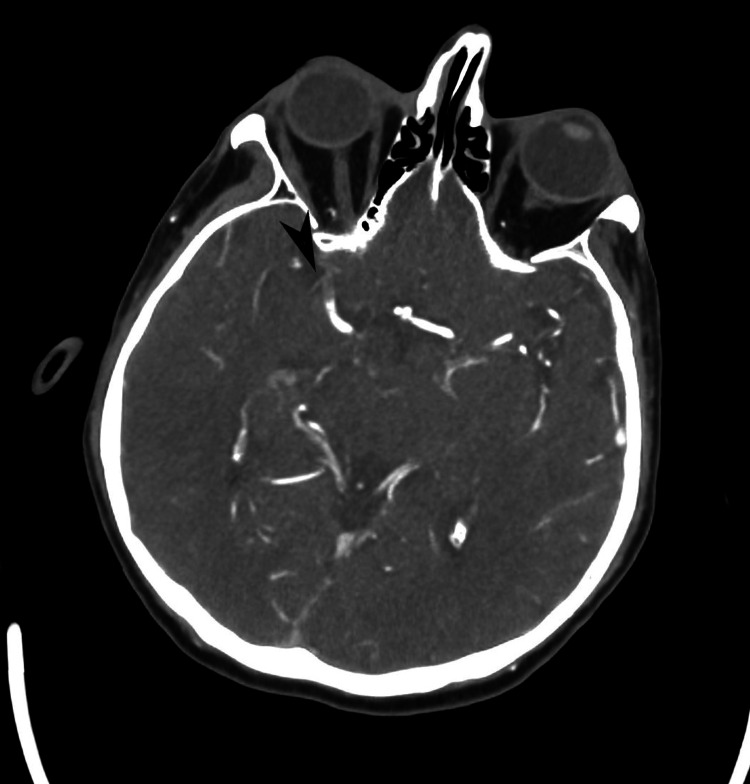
Axial image from subsequent computed tomography angiography (CTA) demonstrating a thrombus within the right middle cerebral artery (M1 segment) (black arrowhead).
